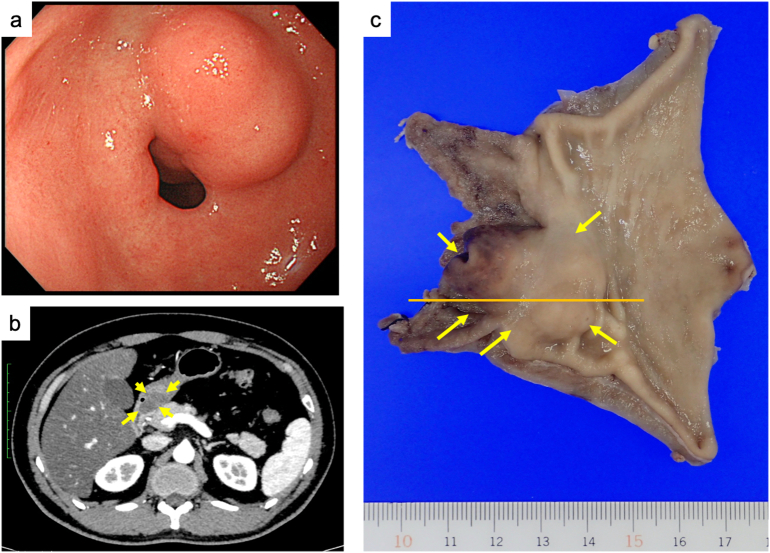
Gastrointestinal endoscopy revealing a submucosal tumor extending from the gastric pylorus through the duodenal bulb (a). Abdominal contrast-enhanced computed tomography showing a pyloric ring submucosal tumor measuring 33 × 19 mm (b). Gross pathology of the lesion (c). The orange line indicates the sectioned surface shown in Fig. 2. Yellow arrows indicate submucosal tumors in (a) and (c). (For interpretation of the references to colour in this figure legend, the reader is referred to the web version of this article.)
